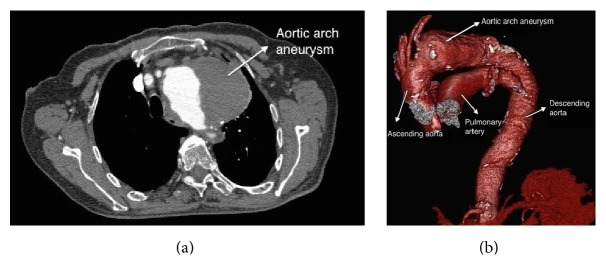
(a) Computed tomography (CT) angiography view of 10 cm diameter aneurysm which includes zone 2 area. (b) Computed tomography (CT) angiography view of 10 cm diameter aneurysm which includes zone 2 area.
